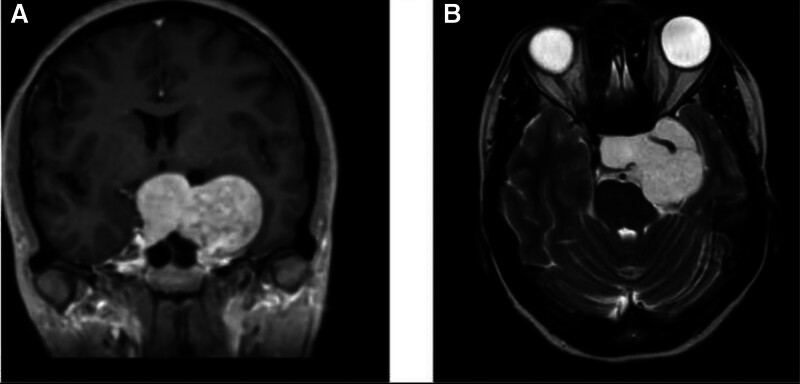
MRI scan of the mass including coronal T1 post-contrast (A) and axial T2-weighted images (B) demonstrating a well-defined extra-axial sellar and left parasellar mass with avid homogenous enhancement encircling the left internal carotid artery. MRI = magnetic resonance imaging.
